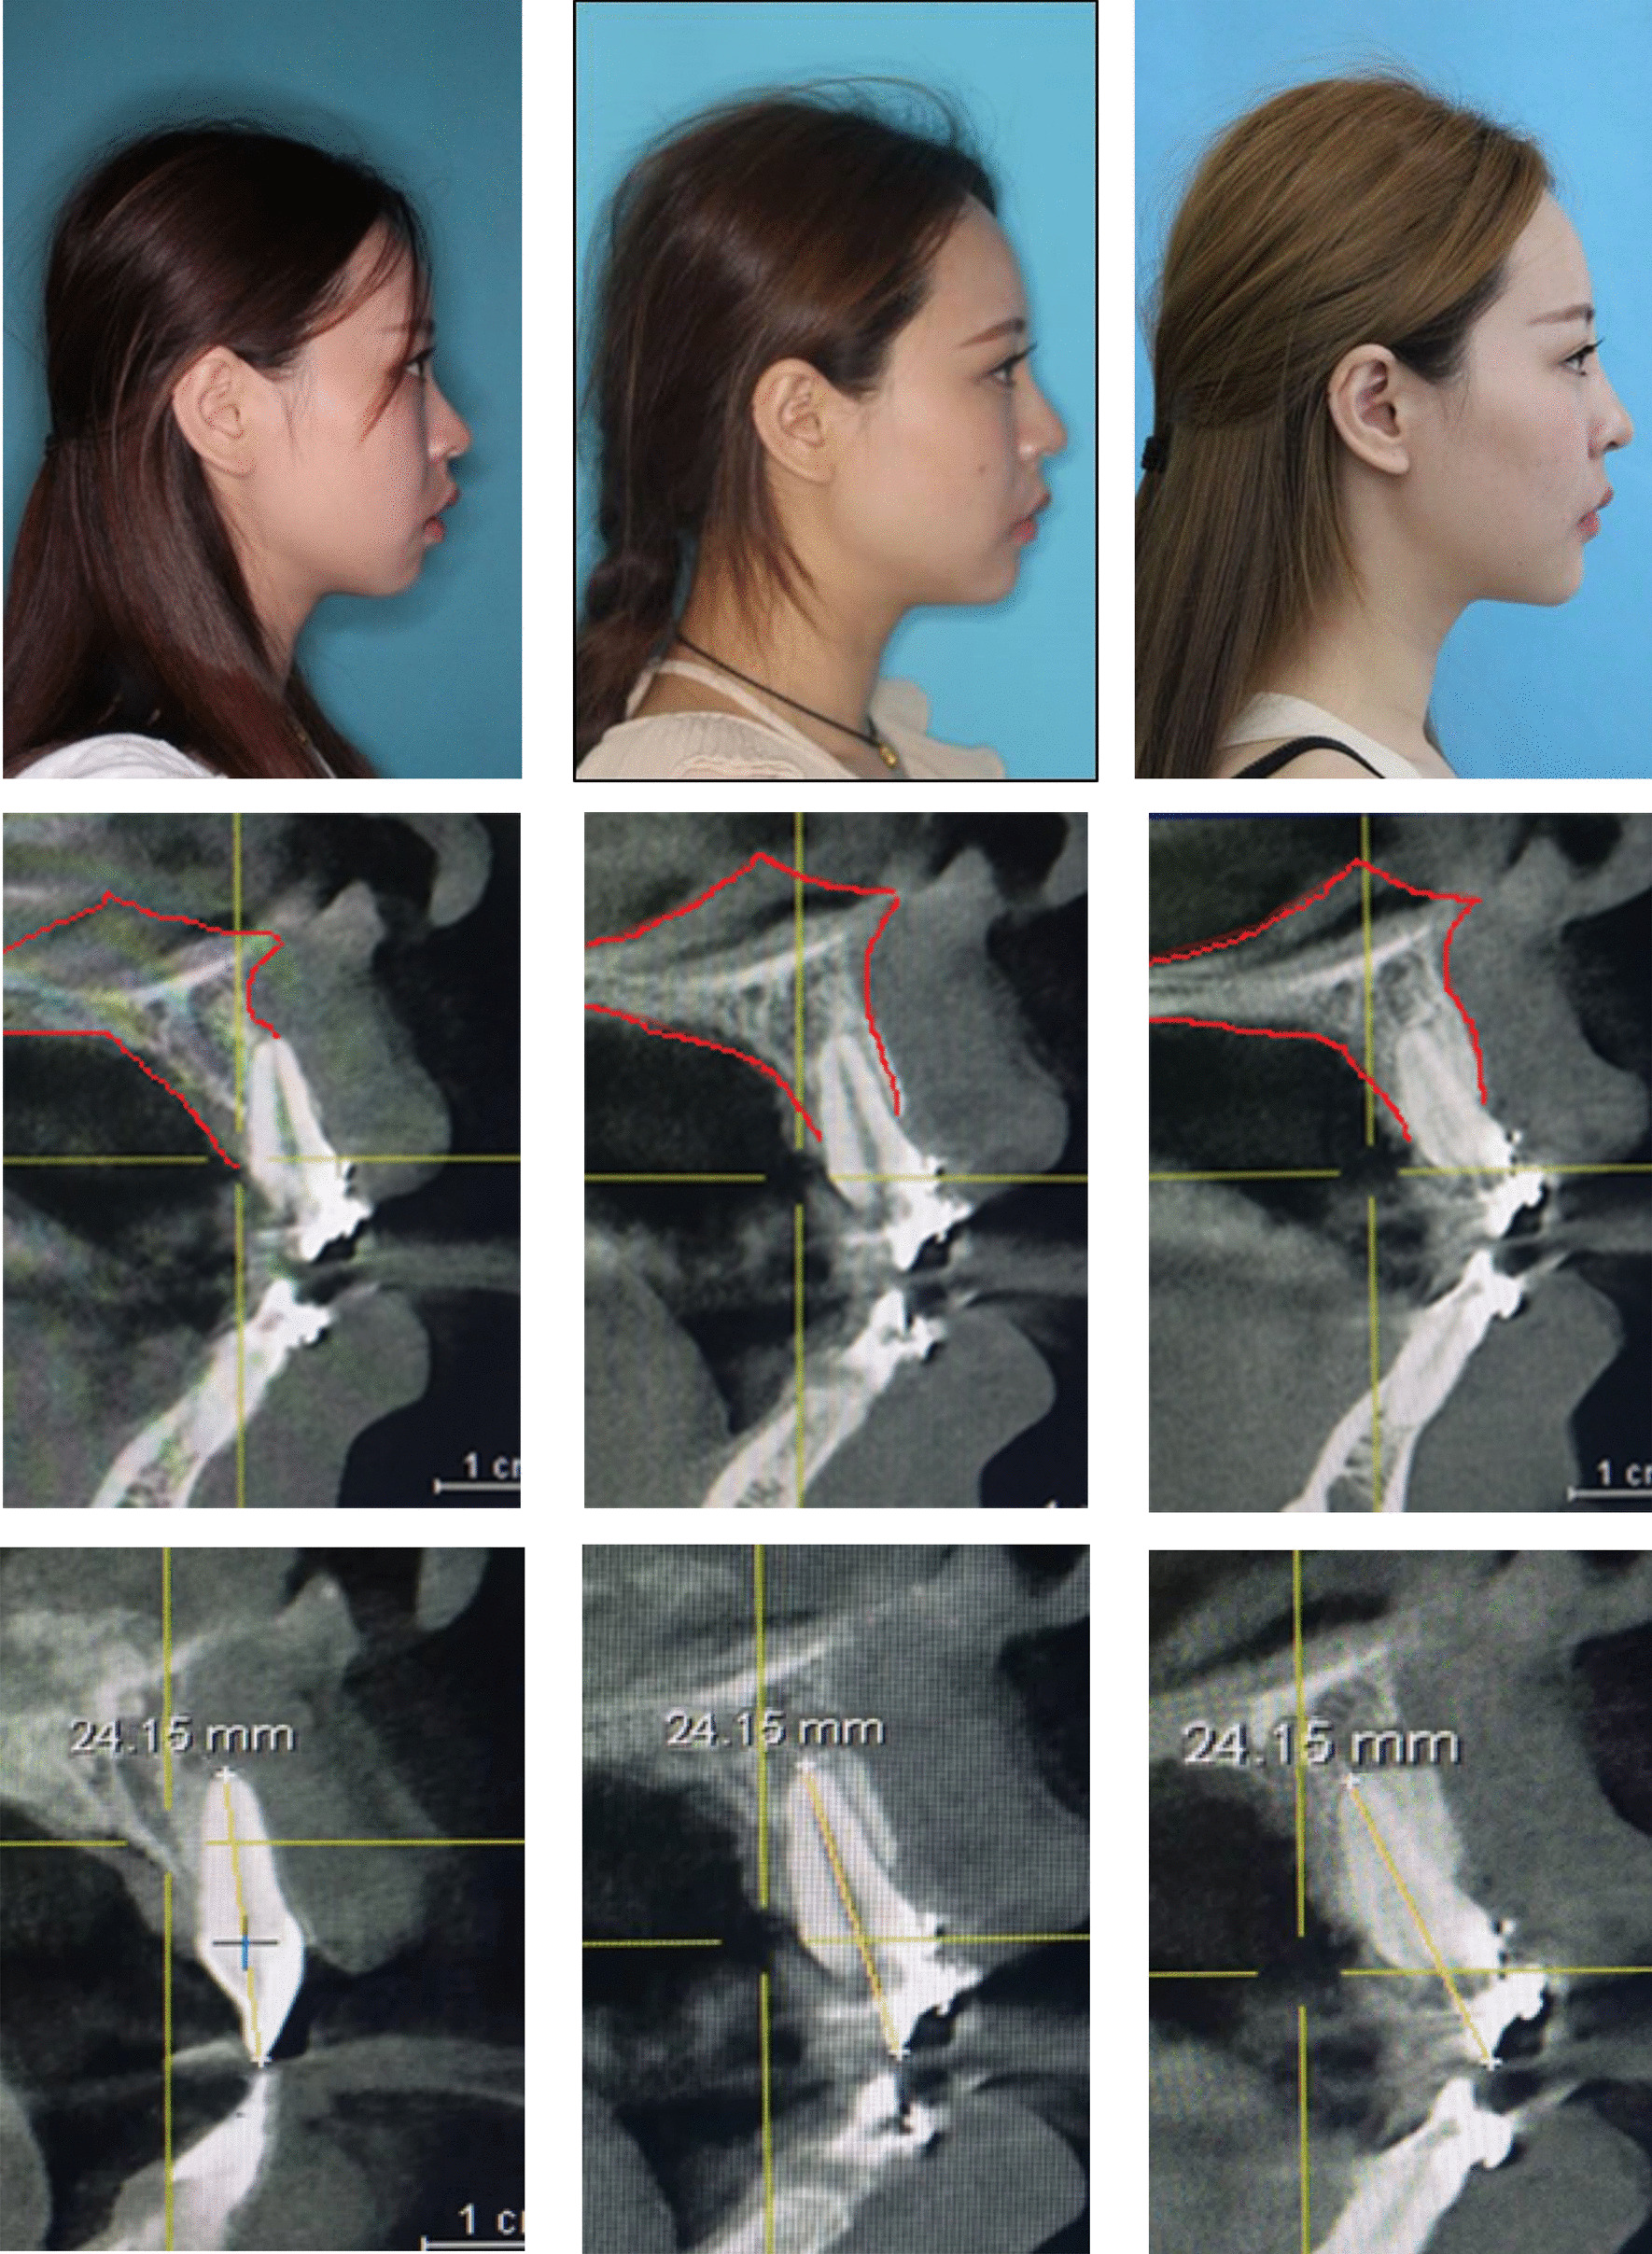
CBCT show tooth root torque change & Root length & Lateral photographs
